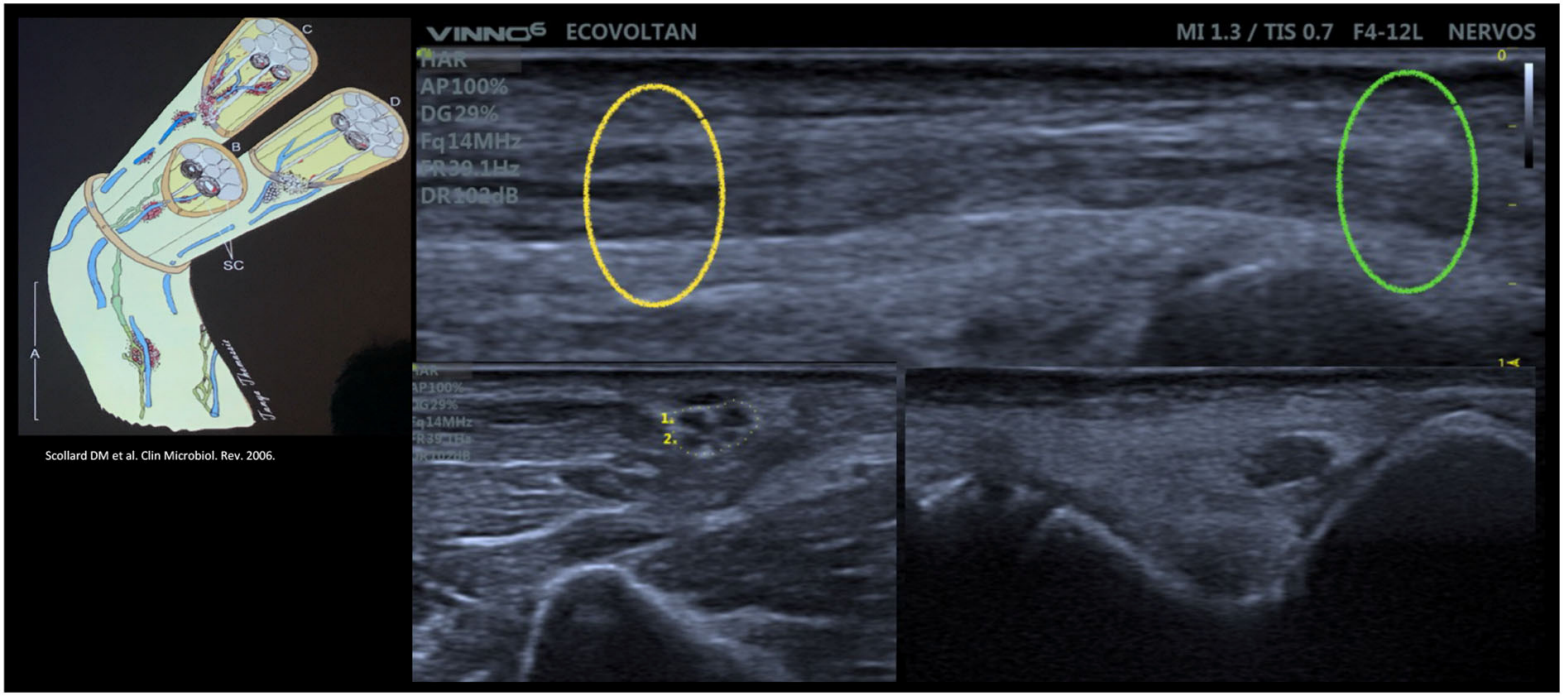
Intraneural focality pattern [Scollard pattern (4) demonstrated by Voltan, G and Frade, MAC with the high-resolution ultrasound] of the ulnar nerve on the longitudinal view (up) and transversal view (down right and down left) showing the different fascicle patterns in the same nerve.
